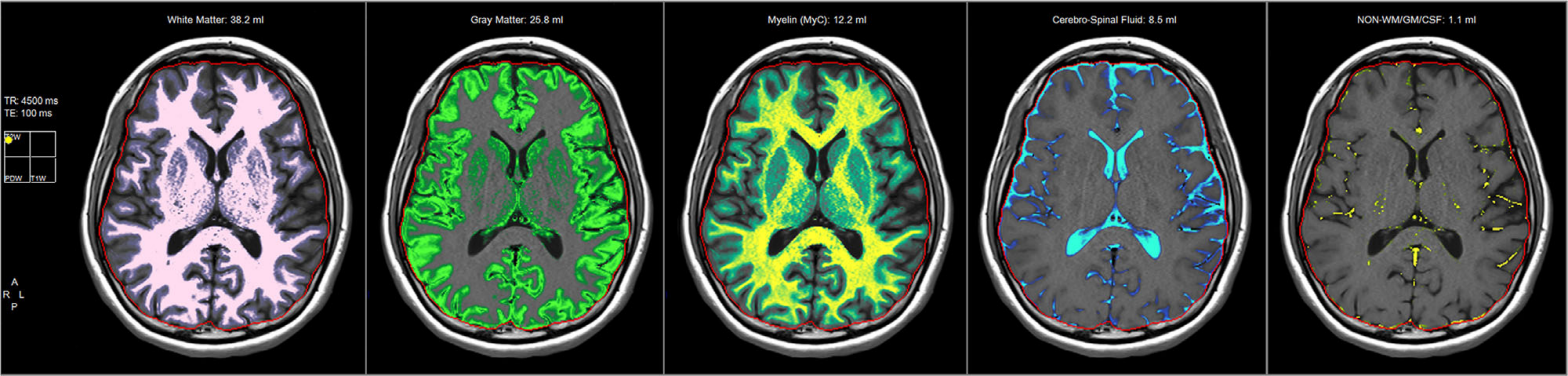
For every individual, the intracranial volume was segmented using a dedicated Synthetic MRI tool. The software created the following segmentations: white matter, gray matter, myelin, CSF, and NON (i.e., non‐white matter, non‐gray matter, and non‐CSF)
